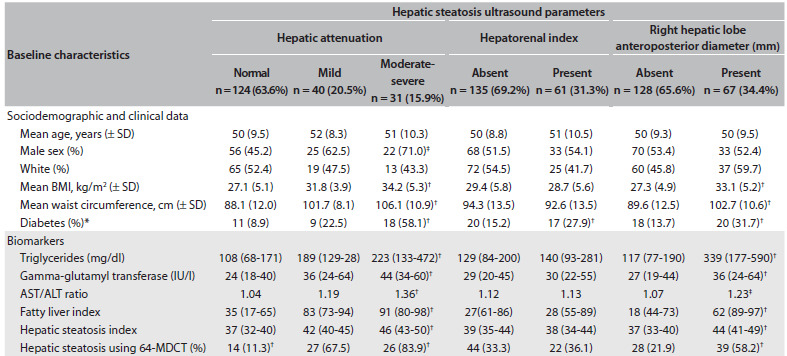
Characteristics in 195 participants from the ELSA study according to sonogram parameters to identify hepatic steatosis
